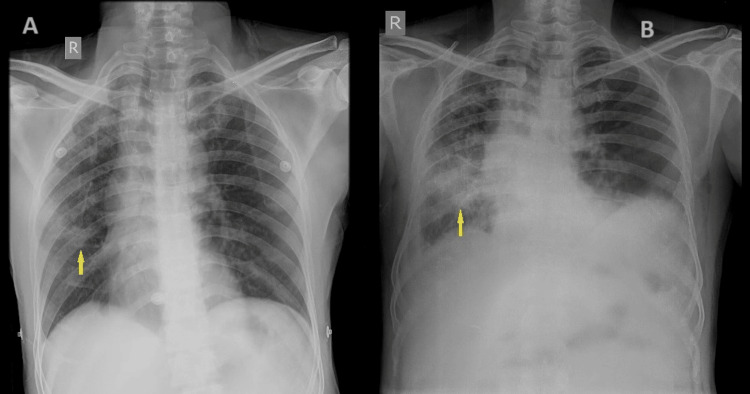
Chest X-ray A. Chest X-ray post-treatment for PTB done in 2019 shows right apical and mid-zone patchy changes of fibrosis. B. Large patch of consolidation in the right middle and lower lung zones showing spiculated margins and air bronchogram within and blunting of costophrenic angles with fissural extension – s/o bilateral pleural effusion.
